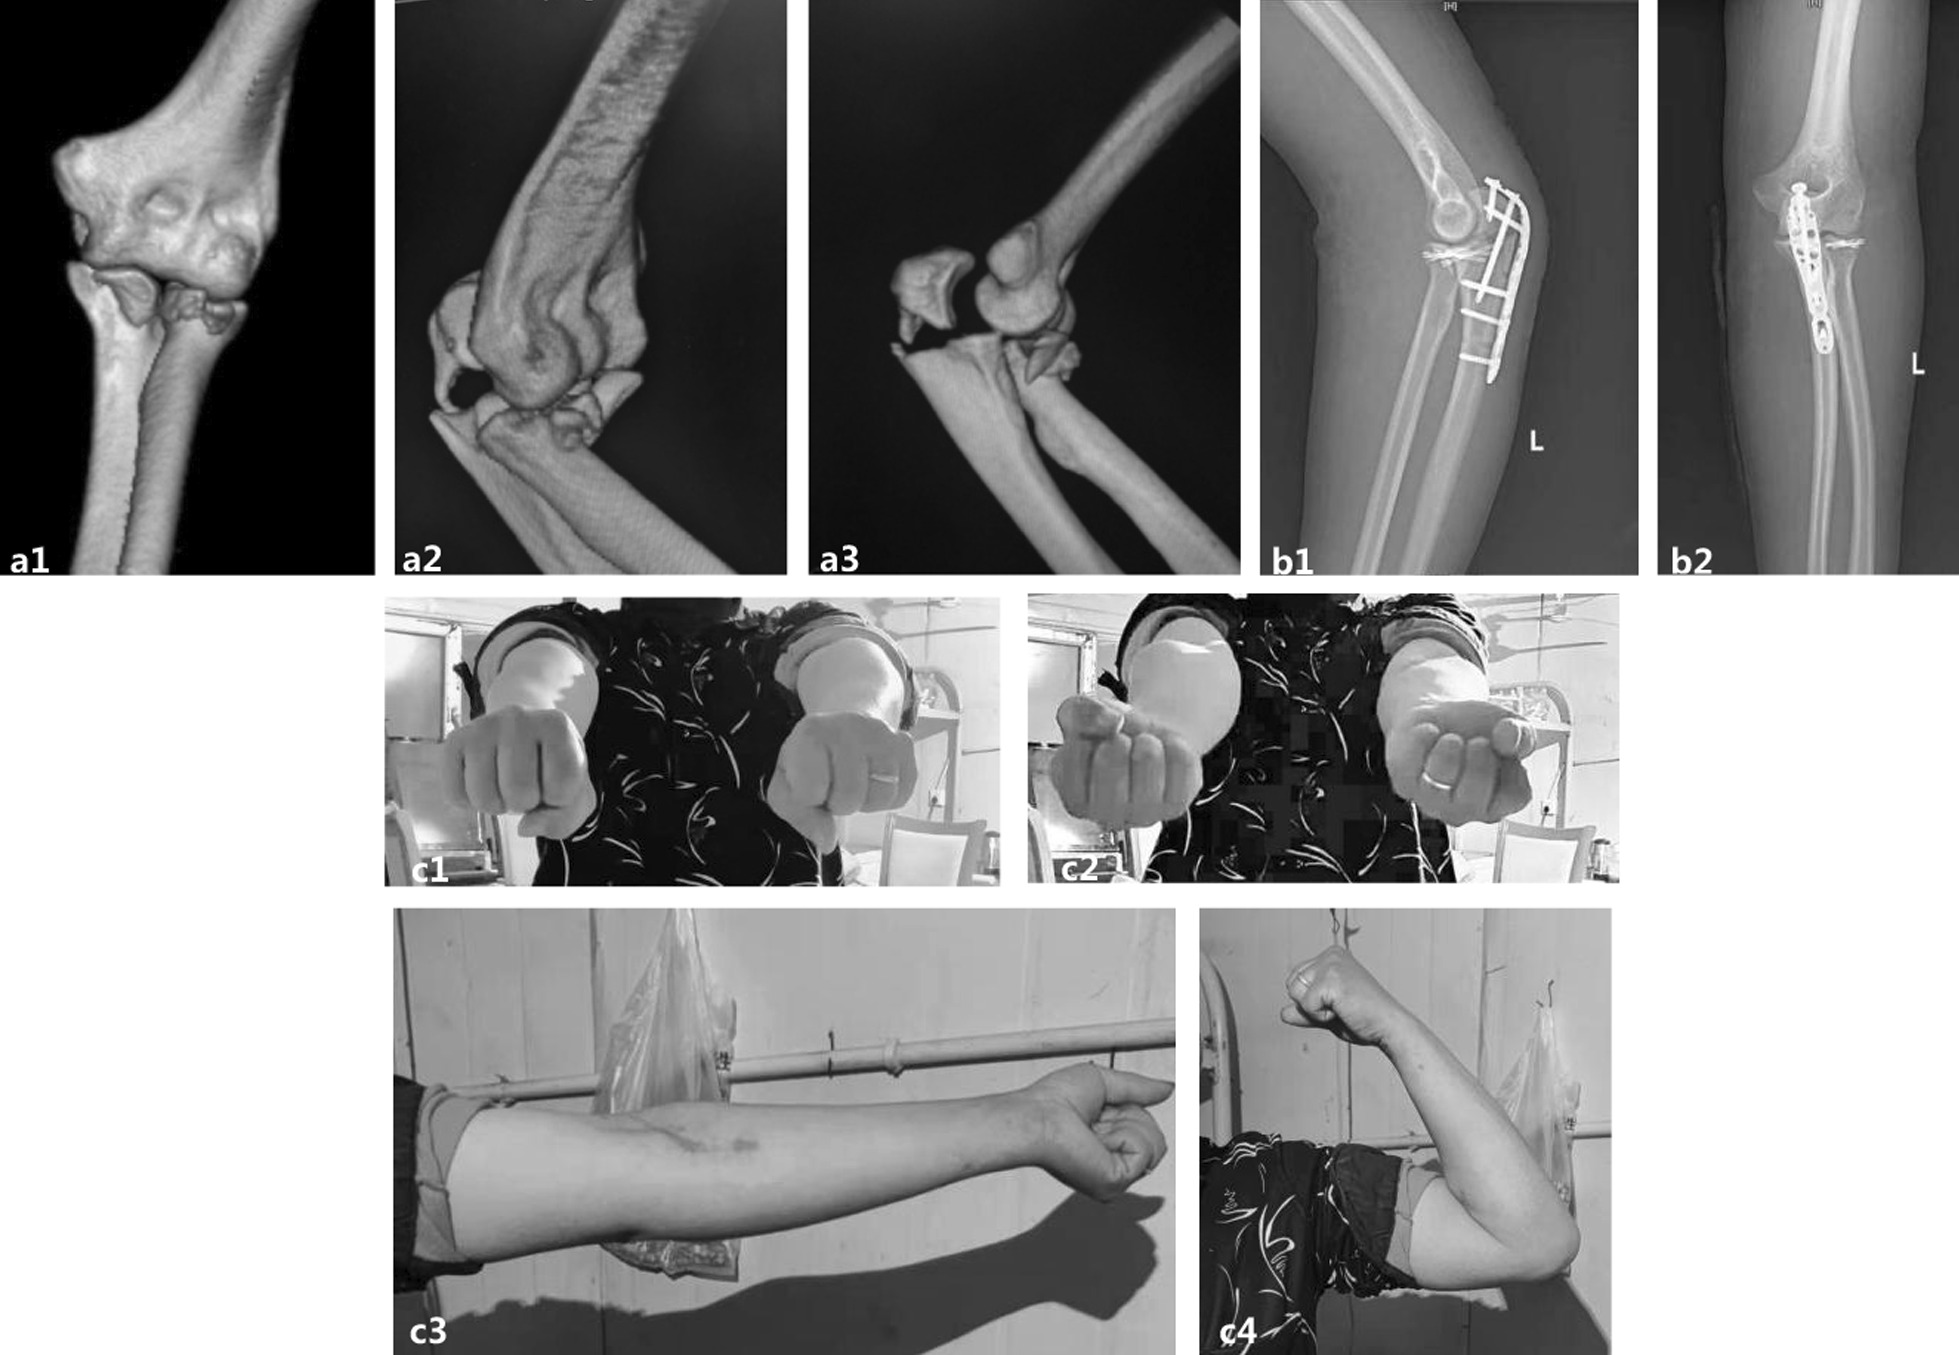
A 37-year-old man presented with a terrible triad of the elbow and an ipsilateral olecranon fracture. Preoperative CT 3D reconstruction (a). Postoperative plain radiographs at one month showed strong internal fixation and good alignment of the fracture (b). Good outcome was achieved at the 1-year follow-up (c)
